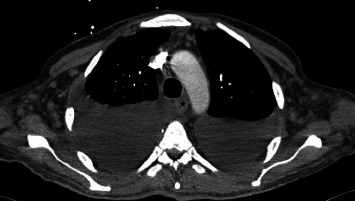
CT of the chest showing mediastinal and axillary lymphadenopathy with bilateral pleural effusions.
